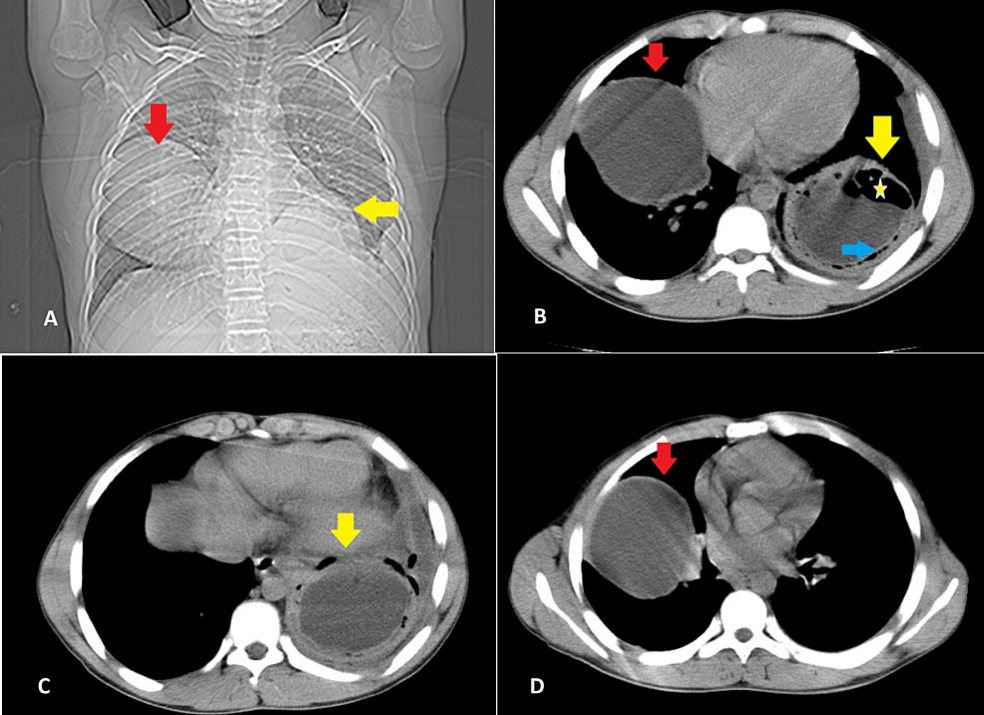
CT Chest CT chest revealed an approximately 8 × 7 cm well-defined cyst in the right mid-zone (panels A, B, and D (red arrow)). Another approximately 8 × 7 cm thick-walled cyst was observed in the left lower zone (panels A, B, and C (yellow arrow)) with an air bubble (panel B, yellow star) and a detached wall (panel B, blue arrow).
